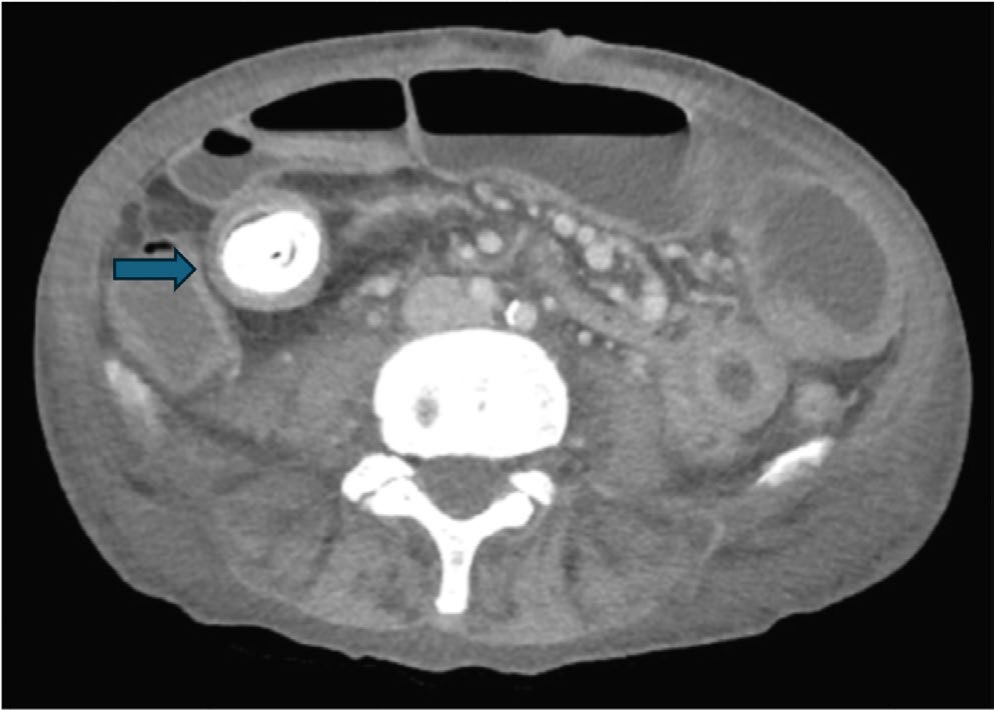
CT scan image demonstrates a stone (blue arrow) impacted in an ileal stricture.
